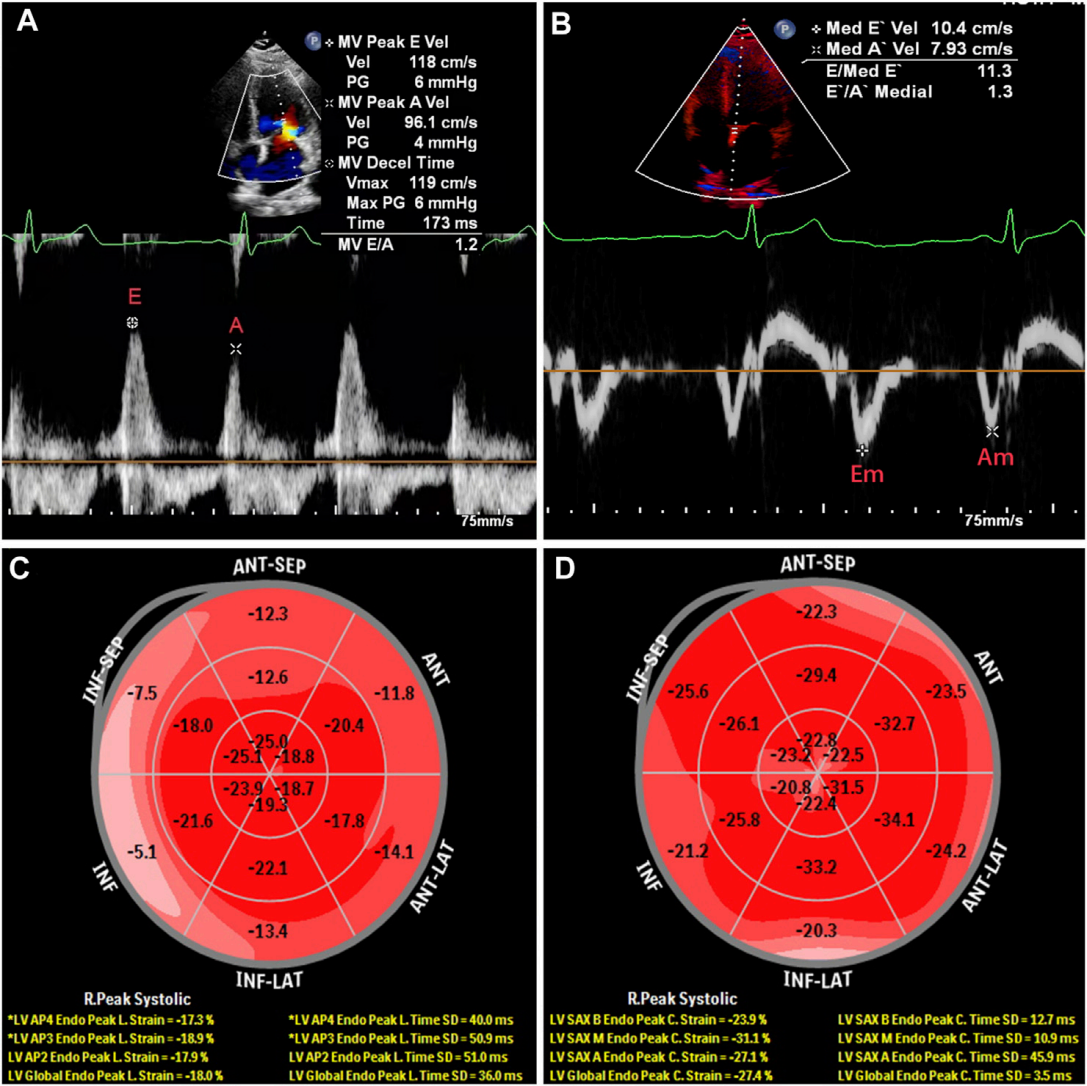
Echocardiography of a 34-year-old male patient diagnosed with gout without secondary hypertension and diabetes. (A), early diastolic peak of mitral valve orifice; (B), the peak early diastolic mitral annular velocity; (C), global longitudinal strain (GLS); (D), global circumferential strain (GCS).
